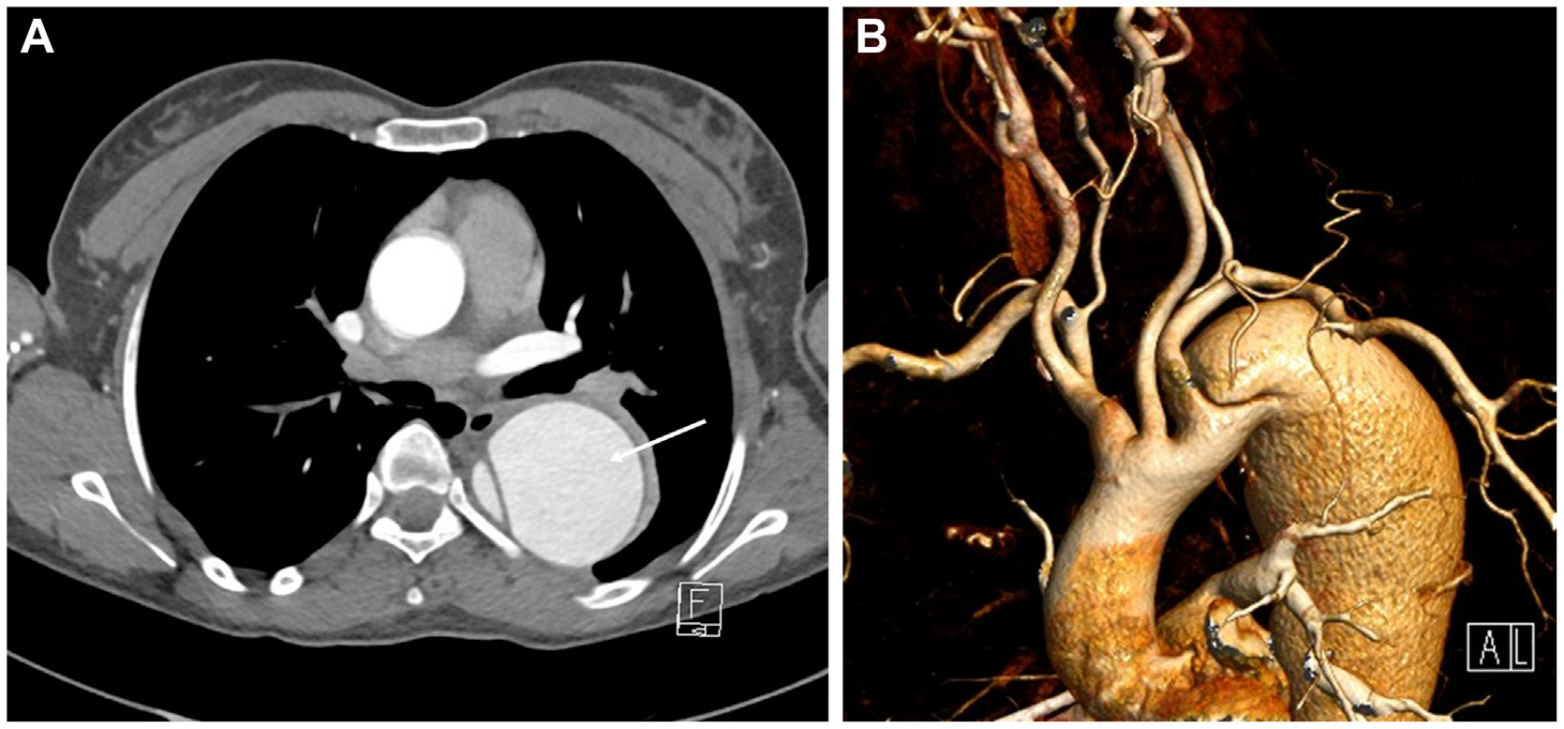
Preoperative computed tomography (CT) arteriogram. (A) Axial CT image of the thoracic aorta. Note the aneurysmal descending thoracic aorta composed of an aneurysmal false lumen (FL) (*arrow*) and narrowed true lumen (TL) (B) Volume-rendered reconstruction. Note the dissection arises immediately distal to the left subclavian artery with crowding of the arteries arising from the arch.
